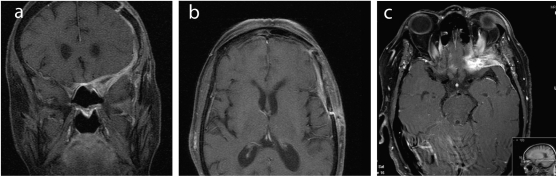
(a-c) MRI images (coronal and horizontal cuts) showing a pachymeningeal thickening around the left anterior clinoid process and lesser wing of the sphenoid, that extends to the left anterior cranial fossa and the anterior part of the middle cranial fossa; it also extends to the orbitary apex through the left optic foramen and superior orbital fissure, affecting the extraocular orbitary muscles, compressing the optic nerve and infiltrating the bone marrow of the lesser wing of the sphenoid and the orbitary wall. This lesion was hypointense on both T1- and T2-weighted images and proeminently enhanced after administration of gadolinium.
